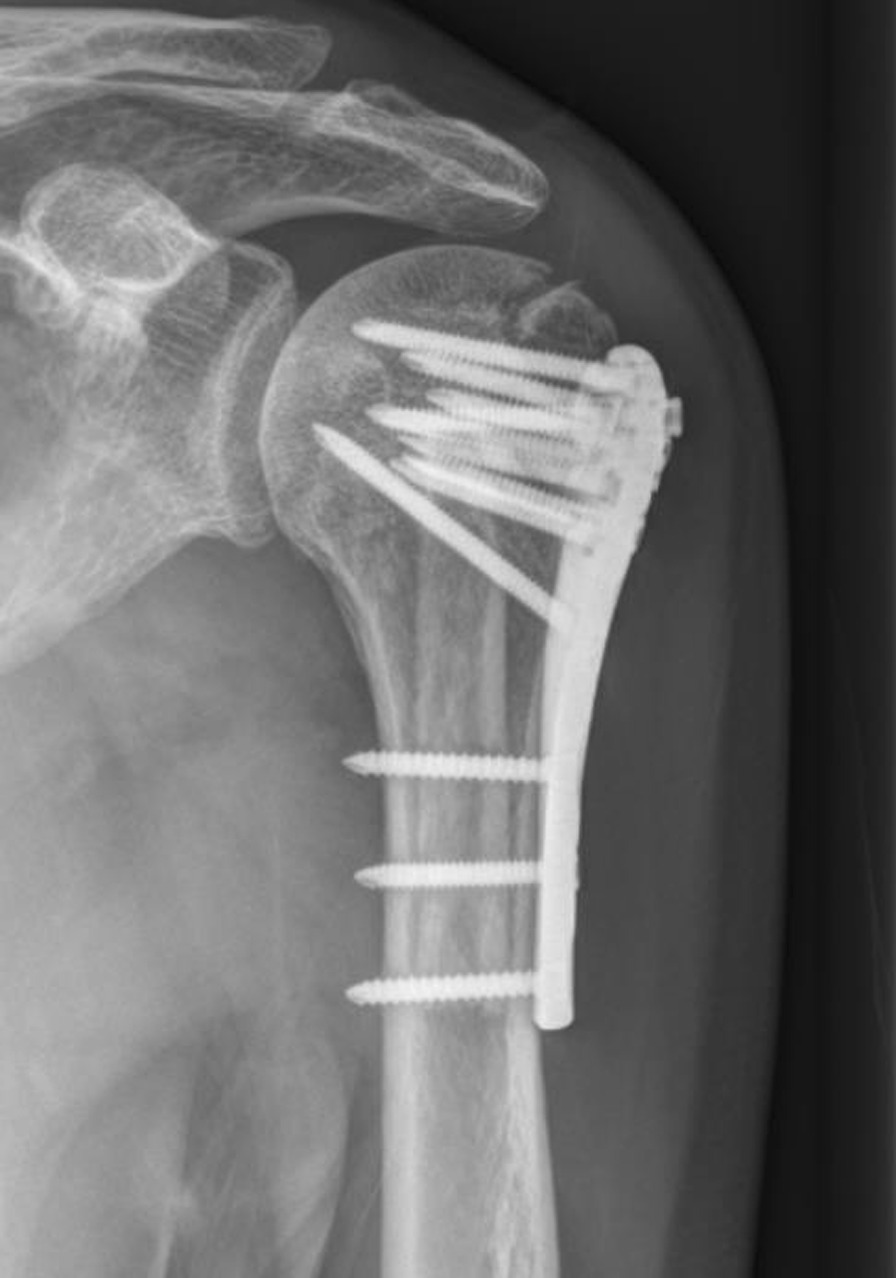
Radiograph for a patient with GT nonunion 12 months after surgery
